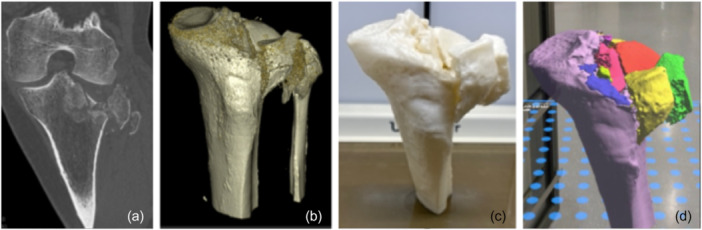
Tibial plateau fracture shown in computed tomography (CT) (a), three‐dimensional (3D) reconstruction (b), 3D printed (c) and mixed reality (MR) reconstructed fracture model (d) imaging modalities.
